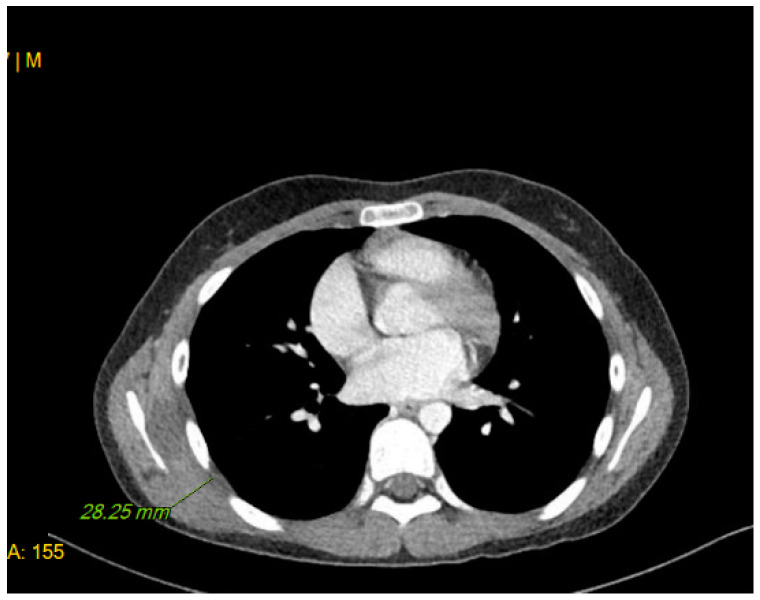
The image of CT scanning of the scapular mass initially presented list of differential diagnosis, elatofibroma dorsi (ED), vascular malformation or other benign soft tissue tumor.
